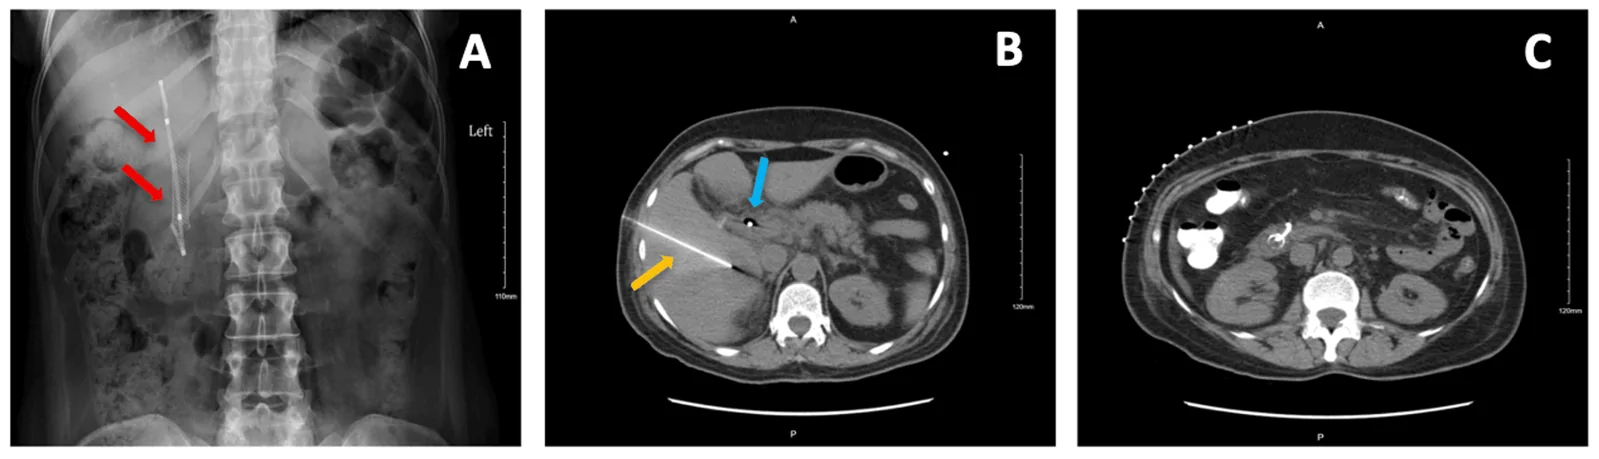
Post-cholecystectomy common bile duct (CBD) and internal biliary stents (red arrows) visualized on abdominal X-ray (A). Under CT guidance, a 21-gauge micropuncture needle (yellow arrow) advanced into the hepatic abscess (B). An ill-defined heterogeneous lesion in the R hepatic lobe with pneumobilia (blue arrow) and a CBD stent is noted (B, C).
